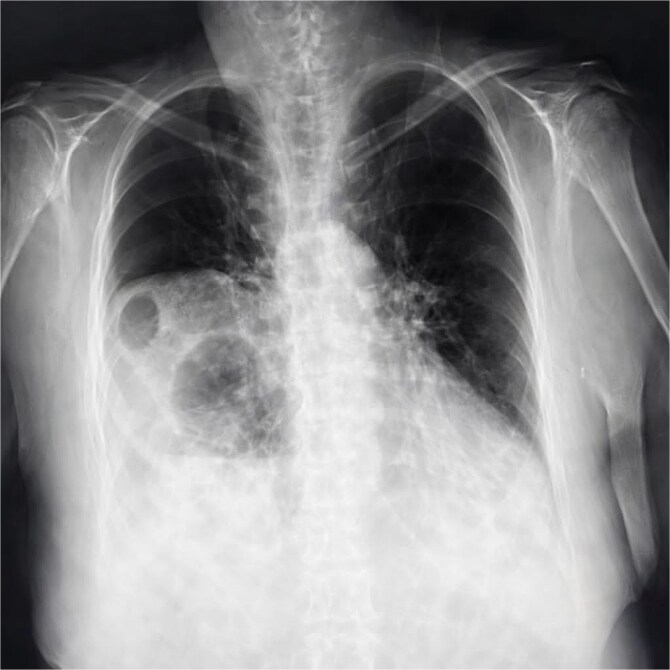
Chest X-ray showing elevation of the right diaphragmatic cupola, eventration (blue arrow), obliteration of the left subdiaphragmatic recess and sub diaphragmatic colonic air fluid level.
